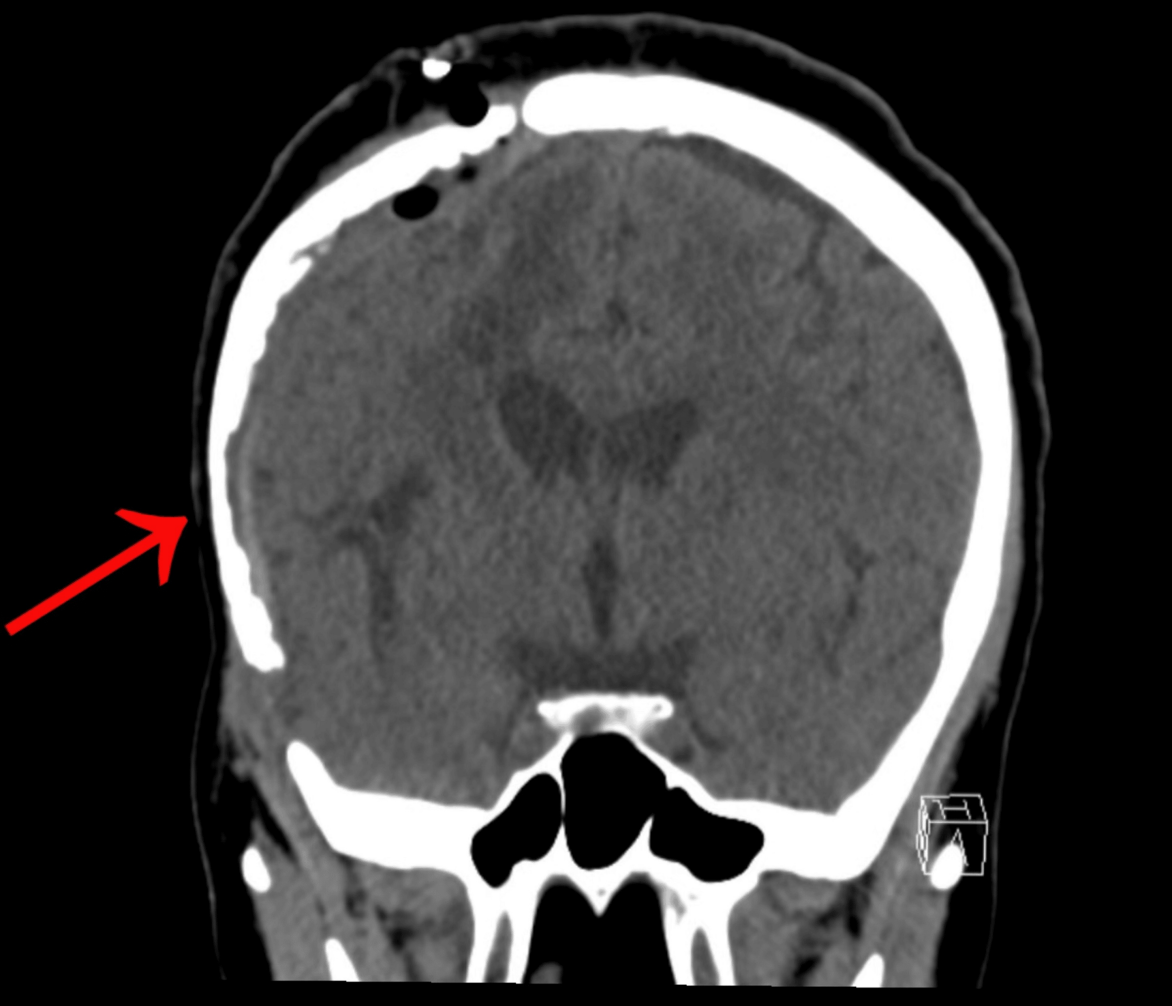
CT head without contrast completed August 2024. Right sided craniotomy with bone flap replacement. Bone flap demonstrating mottled appearance, in line with patient diagnosis of bone flap osteomyelitis, as indicated by the arrow. The epidural space deep to the flap is thickened with an intermediate density matrix, likely secondary to prior debridement the day prior.
